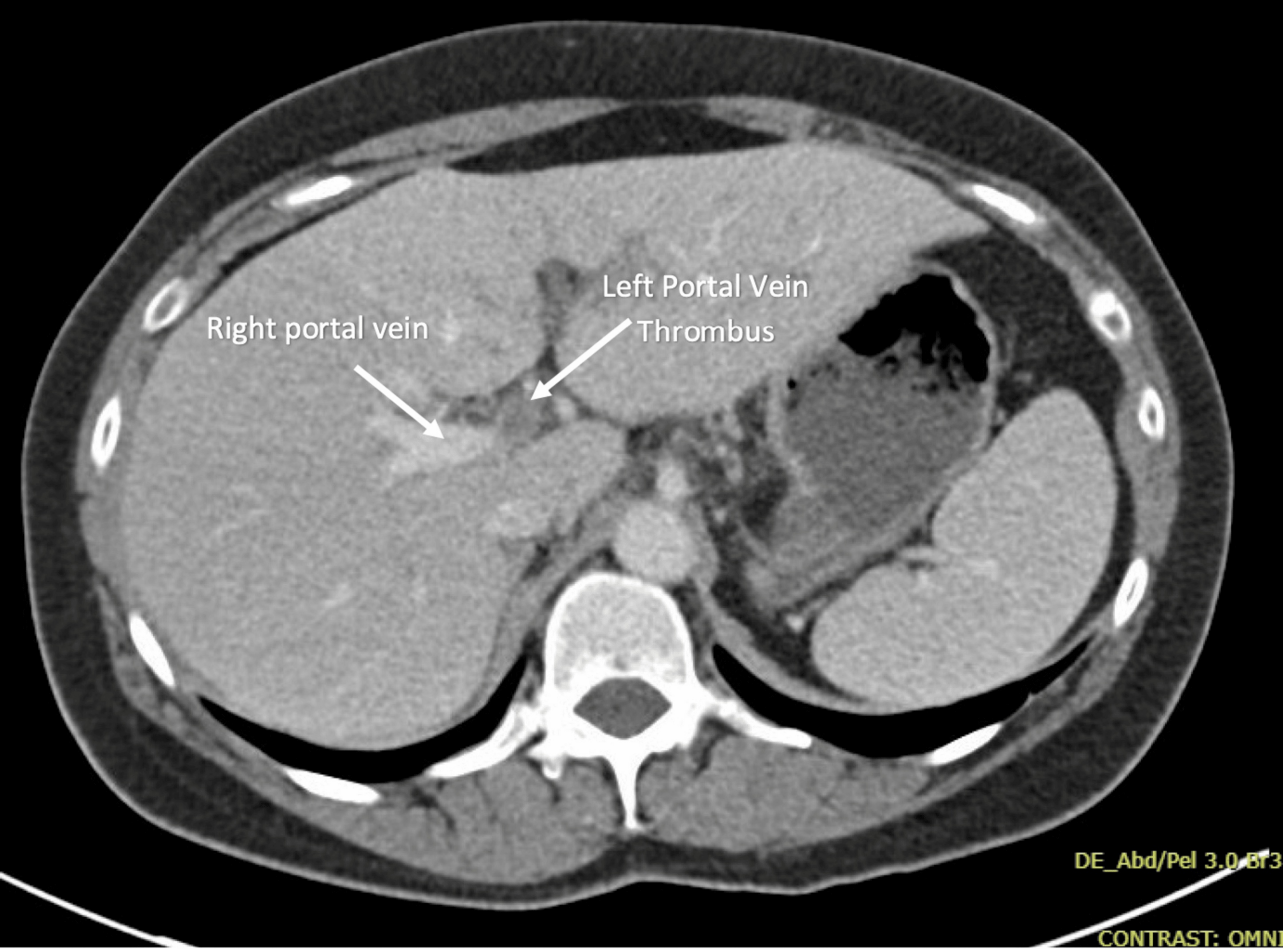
CT abdomen and pelvis with contrast showing a portal vein thrombus. An axial (transverse) CT image shows a filling defect in the left portal vein, consistent with complete thrombosis of the left portal venous system.
